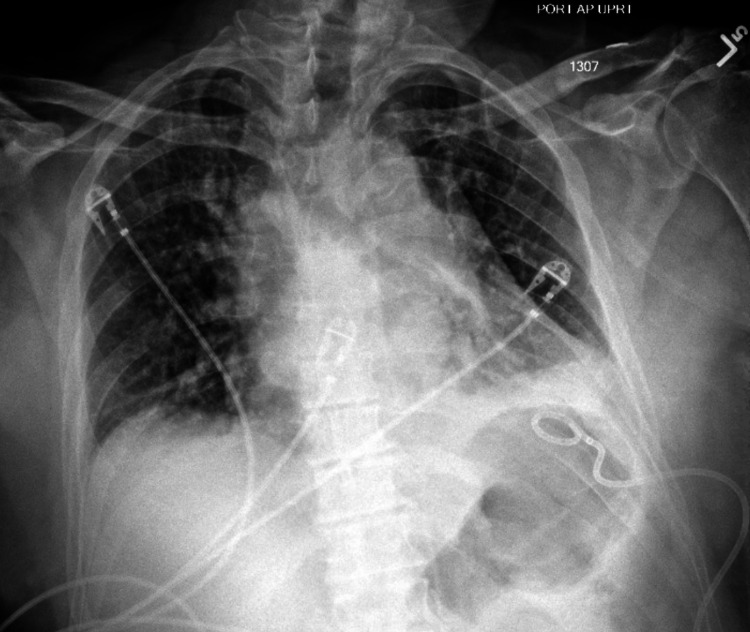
Chest x-ray shows post pigtail catheter placement
